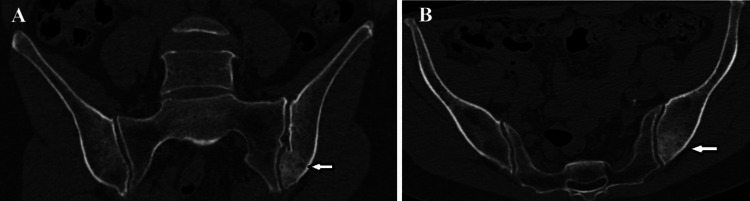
CT scan demonstrating the lesion in the left iliac bone (white arrows). (A) Coronal CT scan showing a sclerotic, ill-defined lesion within the left posterior ilium. (B) Axial CT scan showing a sclerotic, ill-defined lesion with no sclerotic rim in the left posterior ilium.
